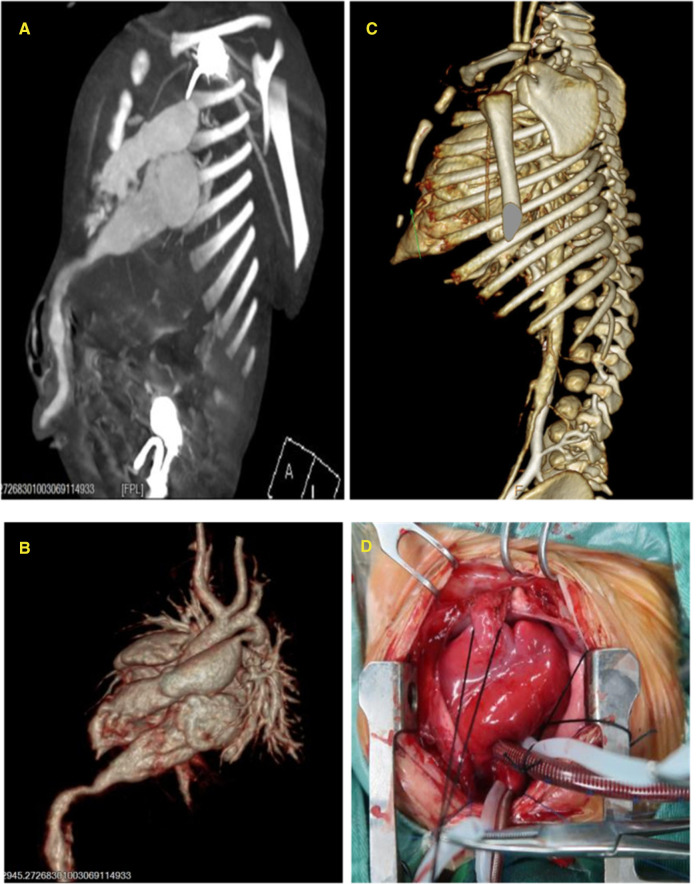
(A) Length of the ventricular diverticulum and narrowed neck connected to the left ventricle. (B) Three-dimensional reconstruction of the left ventricular diverticulum. (C) Partial defect of the lower sternum on computer tomographic angiography (CTA) (arrow). (D) Left ventricular diverticulum seen during operation.
